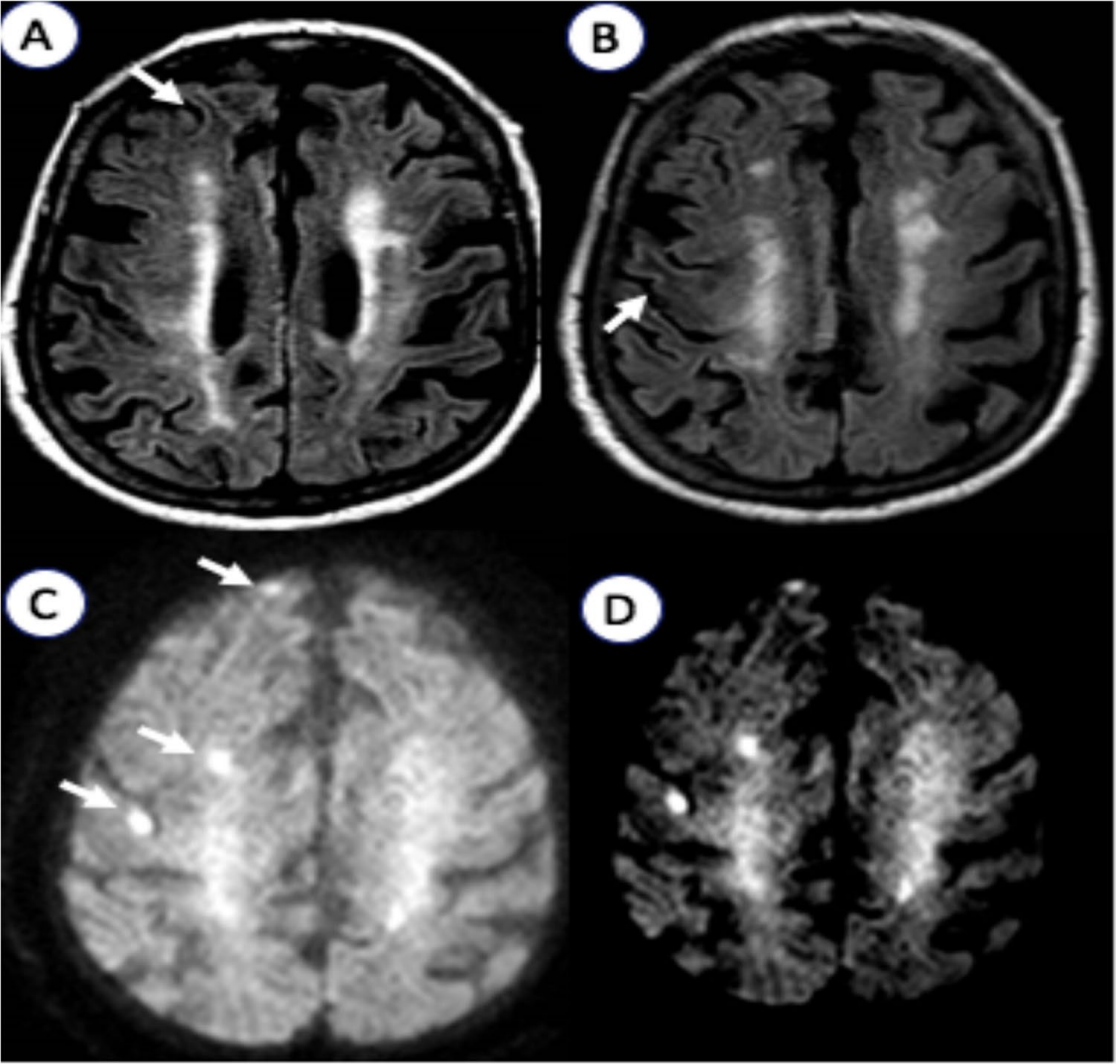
Brain magnetic resonance imaging (MRI). A, B Multifocal right hemispheric subcortical T2-fluid attenuated recovery (FLAIR) hypointensities and C disseminated hyperintensities on diffusion weight imaging (DWI) with D restricted apparent diffusion coefficient (ADC)
